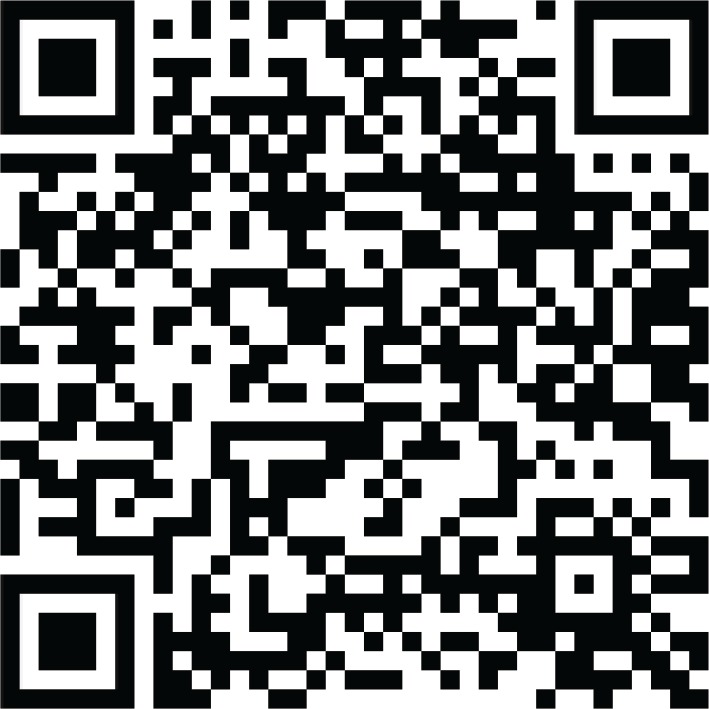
Magnetic resonance imaging, left ventricular long-axis cine imaging: bidirectional turbulent flow.
